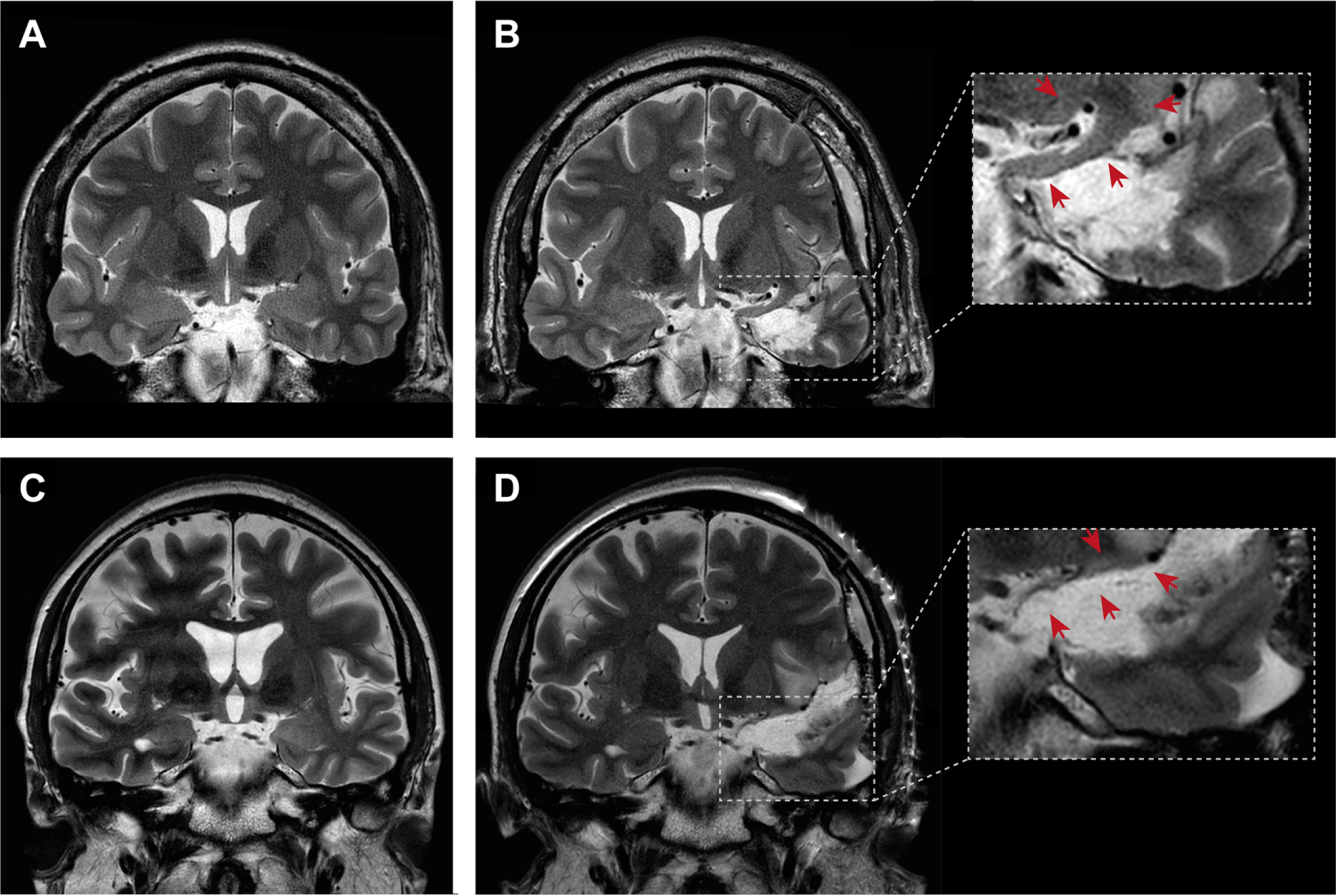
Representative coronal T2‐weighted MRI of tsSAHE with differing extent of piriform cortex resection. Pre‐(A) and postoperative (B) images show profound residual volume of piriform cortex compared to a high extent of piriform cortex resection for respective images (C) and (D). Red arrows in the enlarged sections point at postoperative residual piriform cortices.
